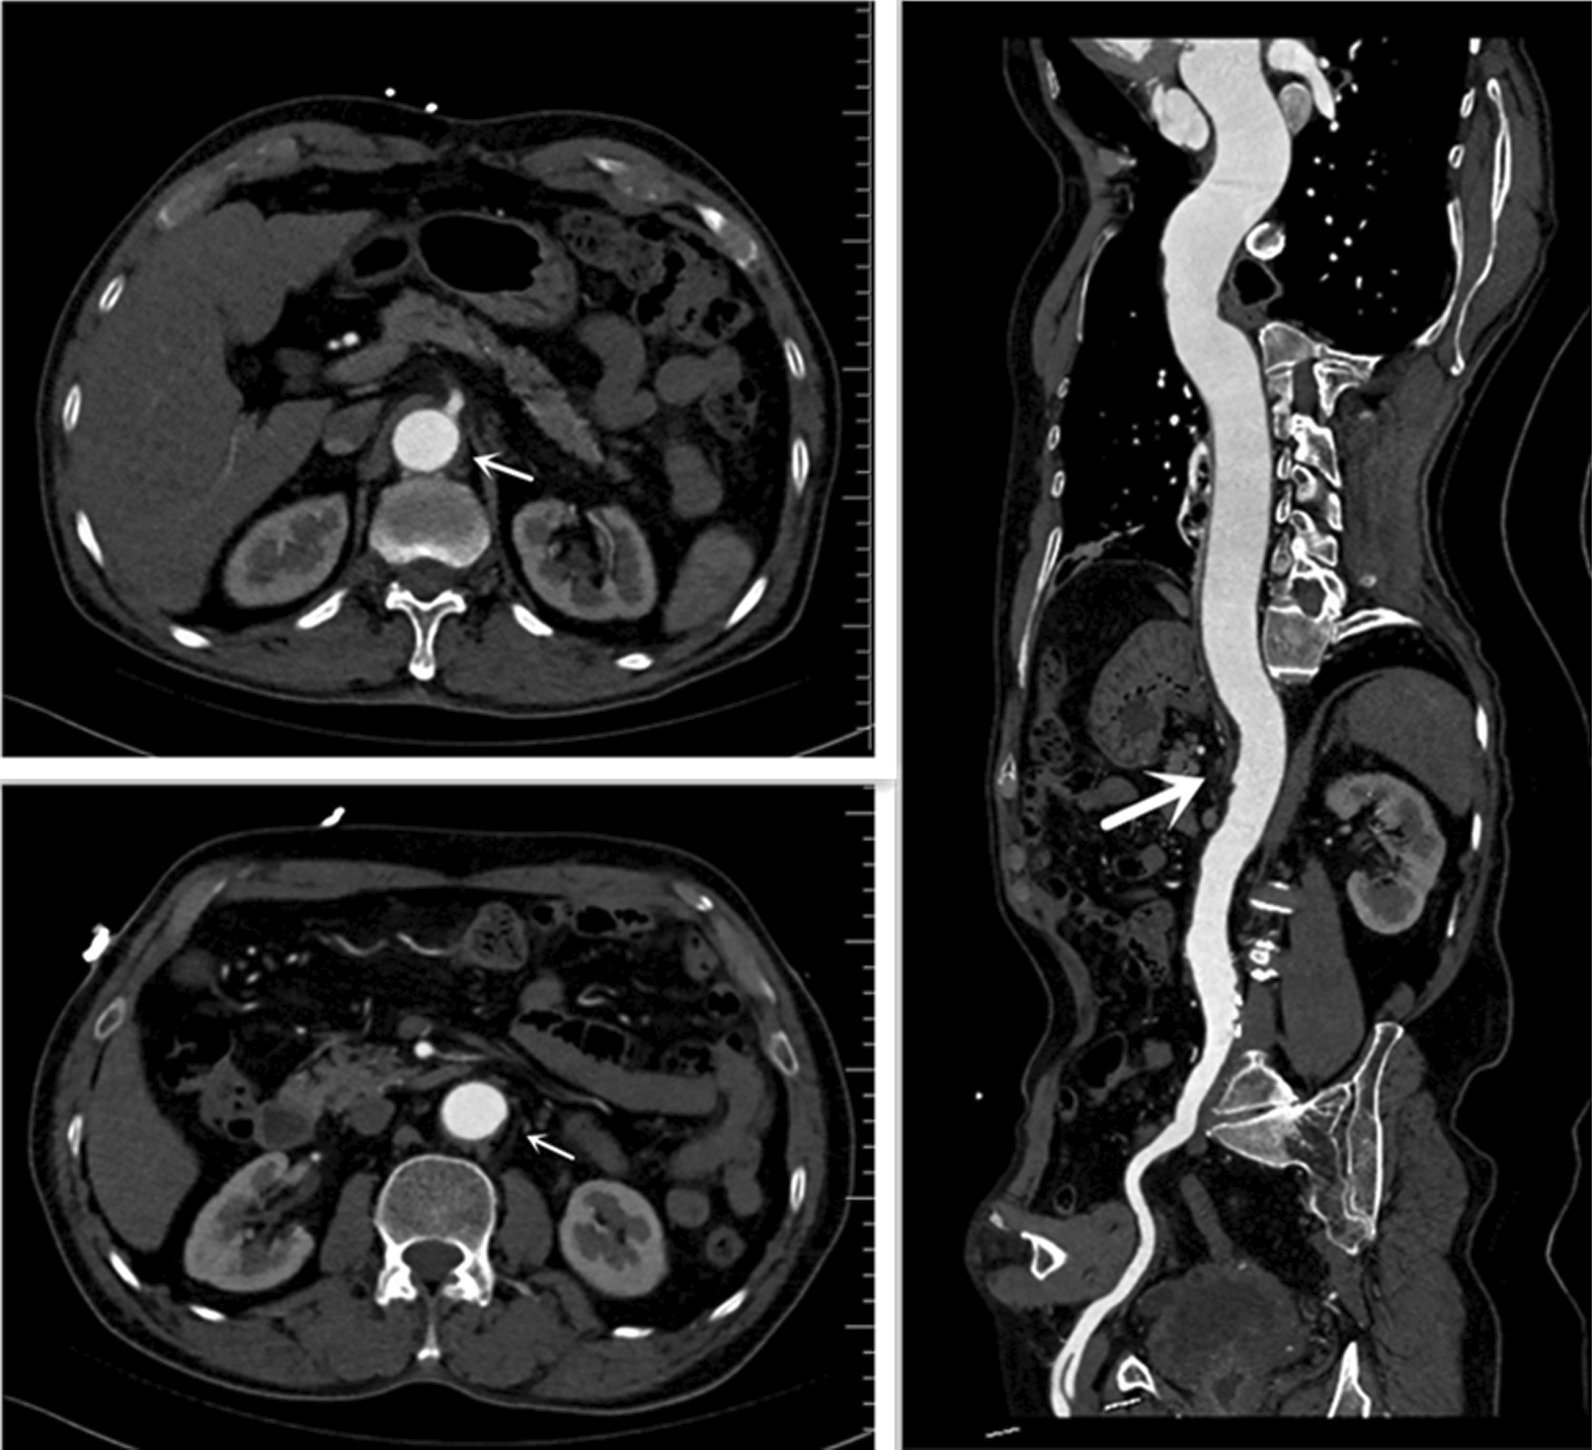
1 year postoperatively, computed tomographic angiography of the thoracoabdominal aorta showed healing of the dissection and the blood vessels were intact. The white arrow indicates that the false lumen at the level of abdominal aorta and Mesentery artery has healed 1 year after operation
